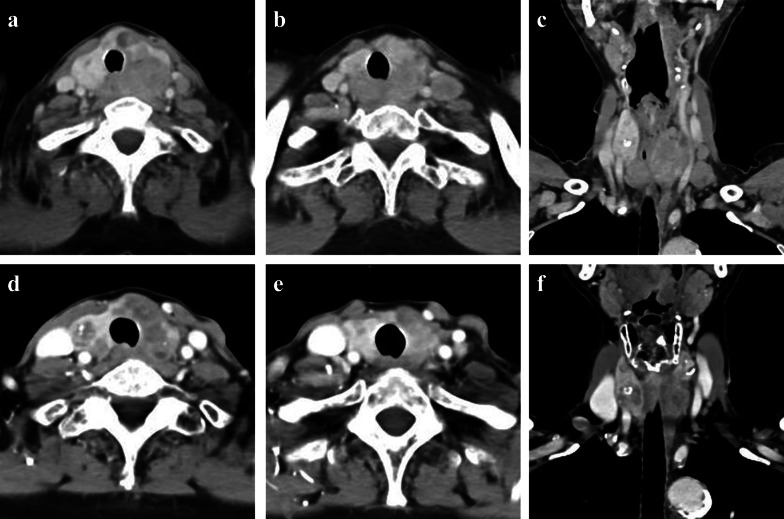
Computed tomography before and after lenvatinib treatment. a–c Computed tomography demonstrated the presence of a thyroid tumor measuring 4.3 × 3.3 cm and left cervical lymph node metastasis measuring 1.9 × 1.6 cm. The thyroid tumor widely invaded the trachea and esophagus. There was no evidence of distant metastasis. d–f. The thyroid tumor and left cervical lymph node metastasis shrank to 3.3 × 2.1 cm and 0.8 × 0.8 cm, respectively, after lenvatinib treatment
